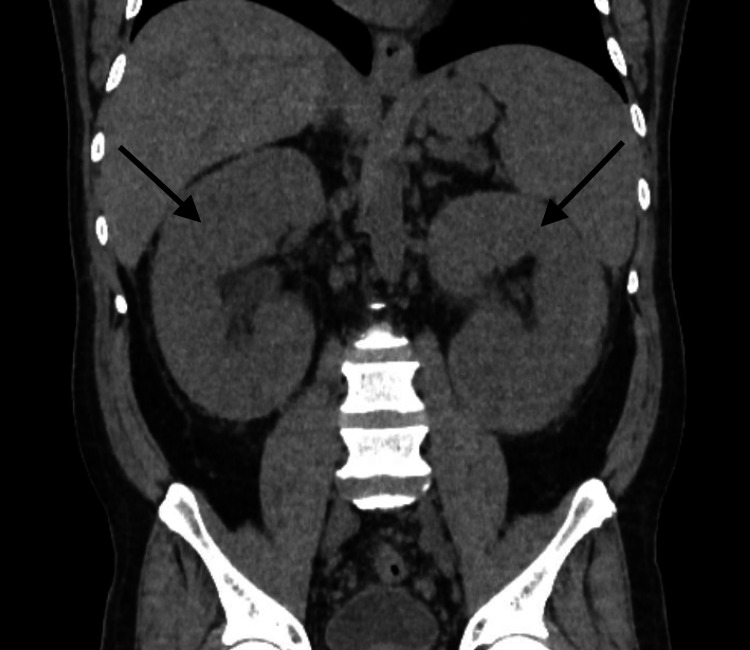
CT abdomen and pelvis without contrast (coronal orientation) demonstrating severe bilateral nephromegaly measuring up to 20 cm indicated by arrows
